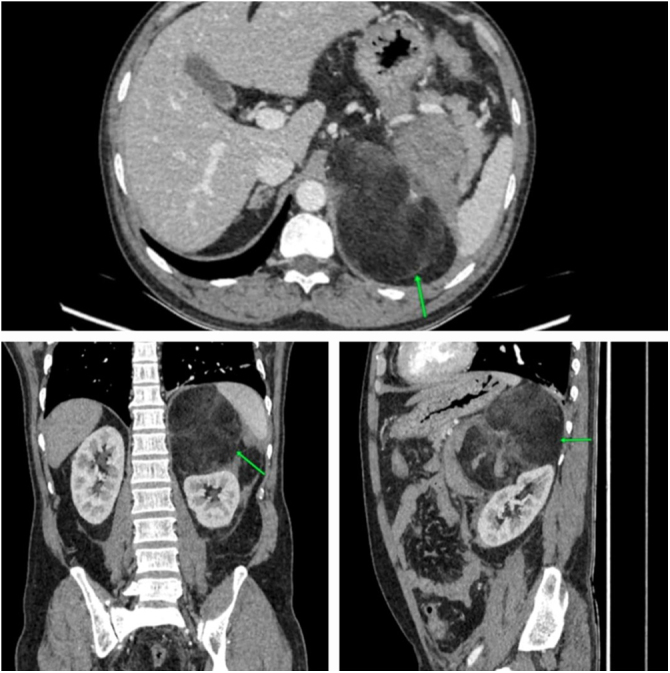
Case 1 – CT abdomen showing left adrenal myelolipoma (yellow arrow) of size 11*10*7.5 cm^3^ in anterior perirenal and pararenal space with non visualization of left adrenal gland separately, superolaterally abutting the spleen, inferiorly abutting and displacing left kidney, anteriorly abutting and displacing splenic vein and tail of pancreas,medially abutting the aorta and posteriorly abutting left hemidiaphragm.
